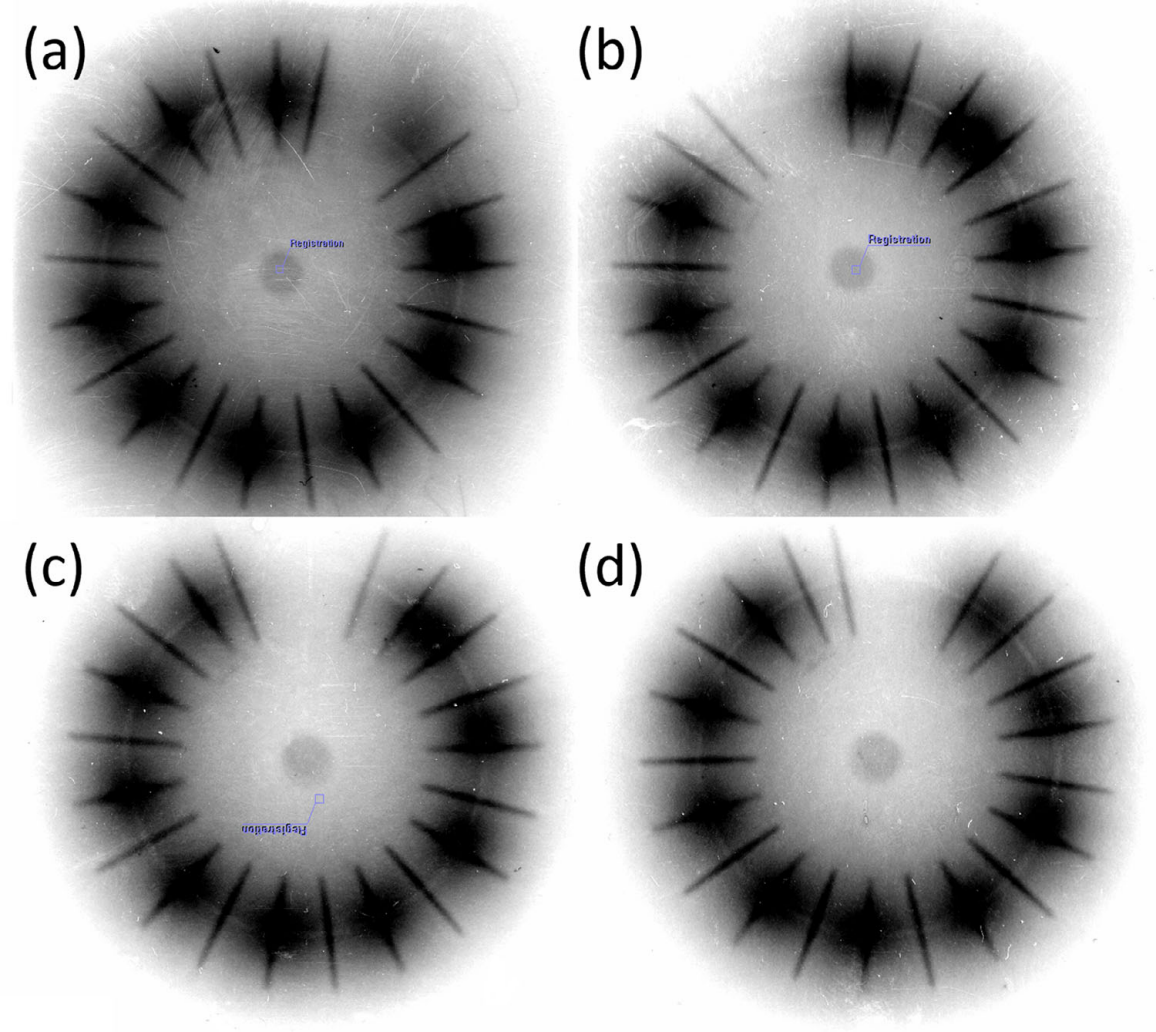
Examples of radiograph using Varian Method ((a) and (b)) and Modified Varian Method ((c) and (d)). (a) and (c) show the odd dwell positions. (b) and (d) show the even dwell positions.
